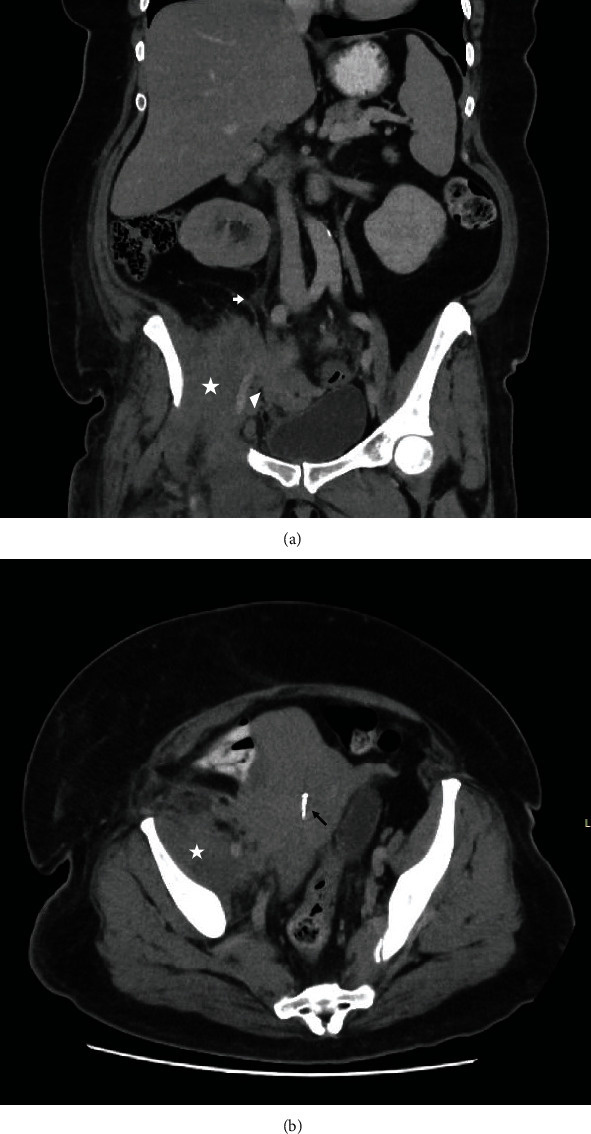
CT (a) coronal and (b) axial of the pelvis, demonstrating a 6 cm phlegmon in the right iliopsoas muscle (marked by star), with extrinsic compression of the right ureter (marked by white arrow), and the right external iliac vein (marked by triangle) and an intrauterine contraceptive device in situ (marked by black arrow).
